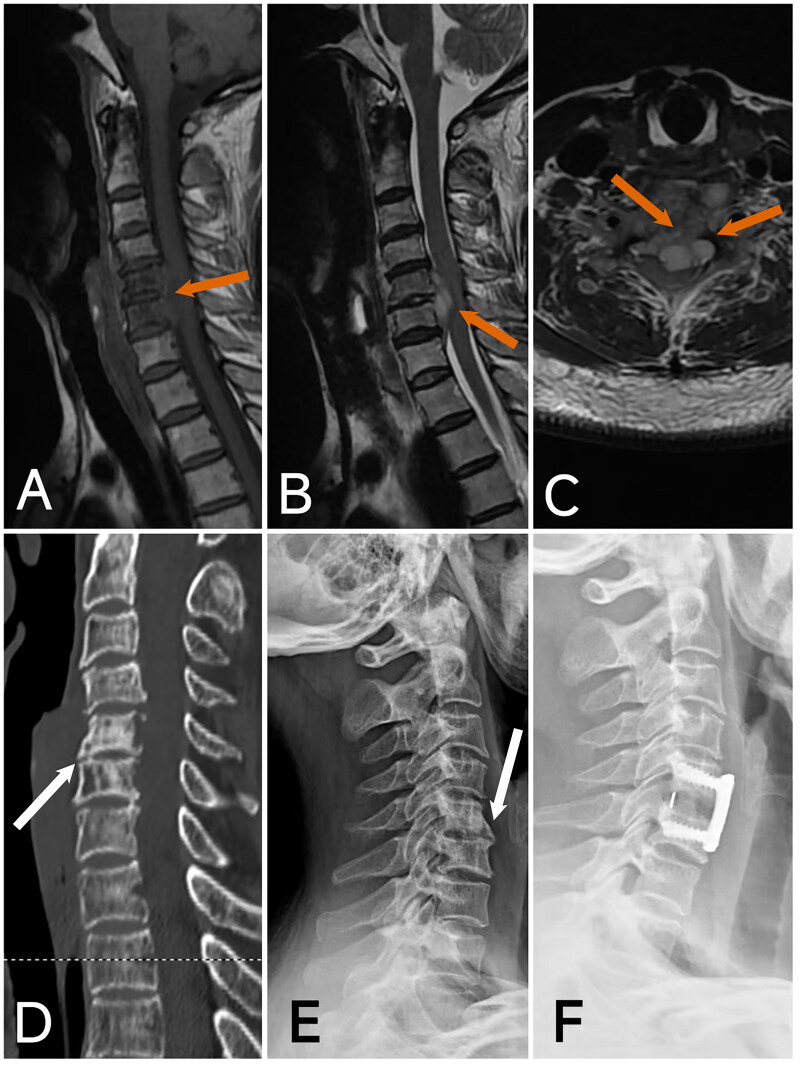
Image of case 6. Case 6: A: T1-weighted imaging (T1WI); B: T2-weighted imaging (T2WI); C: Axial T2-weighted imaging (T2WI) shows an epidural abscess at the C5-6 segment with significant spinal cord compression (red arrow). D, E: CT and X-ray reveal loss of cervical curvature, vertebral instability, mared narrowing of the C5-6 intervertebral space, and "lip-like" changes at the anterior edge of the vertebrae (white arrow). F: Postoperative X-ray at 4 months demonstrates good positioning of the internal fixation, restoration of cervical curvature, recovery of the C5-6 intervertebral height, and fusion of the intervertebral bone graft.
